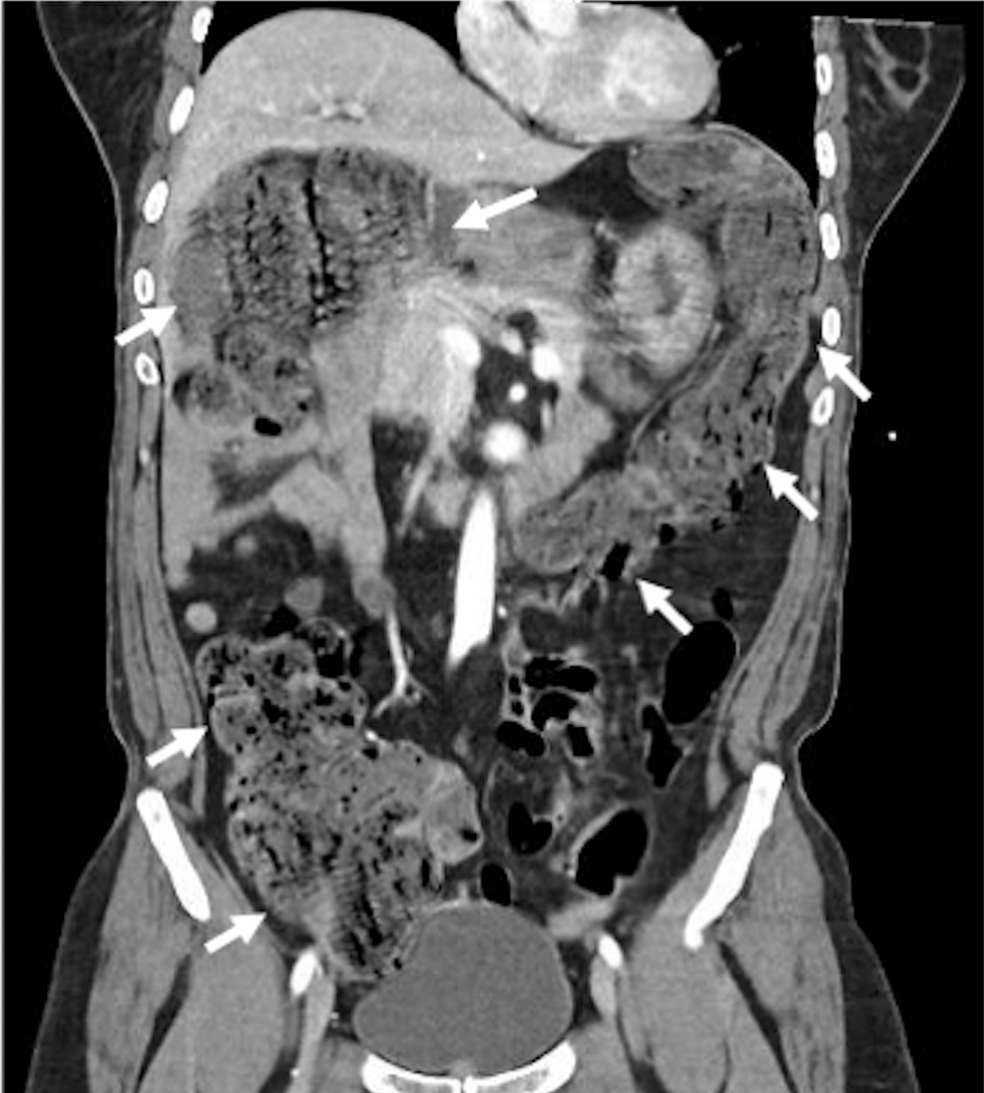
Abdominal CT scan. Arrows demonstrating the extent of a distended colon. An axial scan demonstrated packed feces during the course of the hospital stay, which was suggestive of constipation
